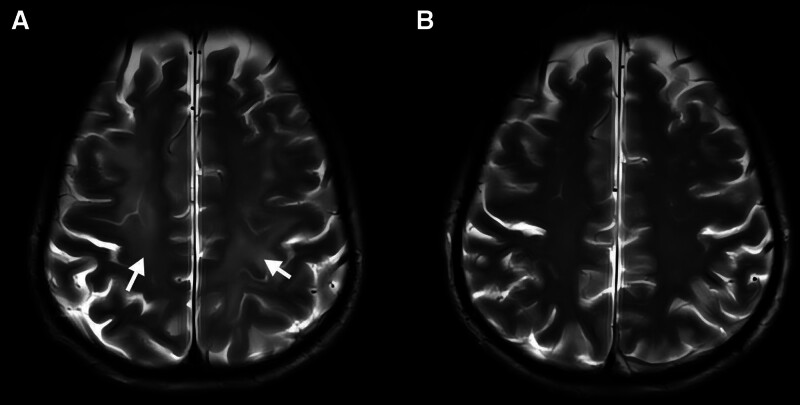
Magnetic-resonance evolution of cerebral injury. (A) Brain MRI on March 5, 2025 (day 8) shows multifocal swelling of the bilateral frontal, parietal and occipital lobes and both cerebellar hemispheres (white arrows). (B) Follow-up MRI on April 8, 2025 (day 43) reveals near-complete resolution of cerebral edema; the previously swollen regions have returned to almost normal signal intensity (white arrows). MRI = magnetic-resonance imaging.
